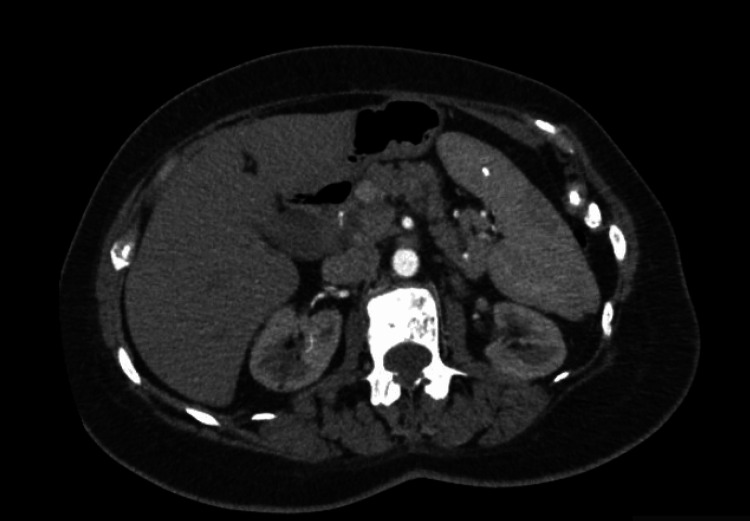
Axial computed tomography depicting metastatic involvement of the liver and pancreas (September 2021).
